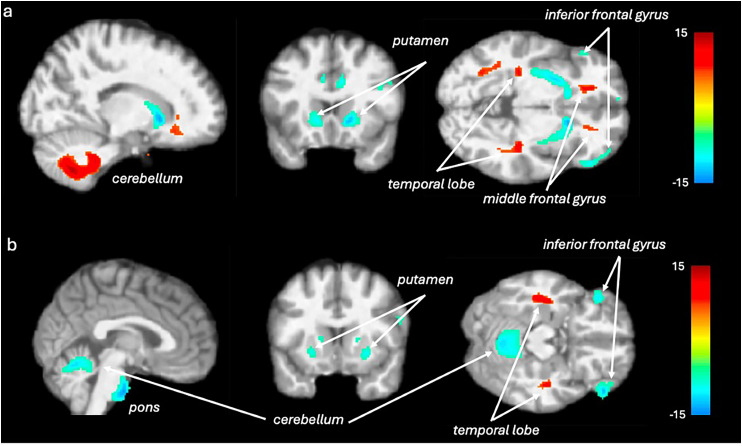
Brain regions with significant metabolic differences in MSA patients compared with healthy controls detected by SPM analysis of FDG-PET scans. a) Normalized glucose metabolism in the MSA-P patients decreased (blue) in the bilateral putamen, inferior frontal gyrus and anterior cingulate, and increased (red) in the bilateral temporal lobe, middle frontal gyrus and posterior lobe of the cerebellum compared to healthy controls (*p* < .001 uncorrected at peak-level). b) Normalized glucose metabolism in the MSA-C patients decreased (blue) in the vermis of the cerebellum, the pons, the bilateral inferior frontal gyrus, and the putamen, but increased (red) in the left and right temporal lobe compared to healthy controls (*p* < .001 uncorrected at peak-level). Only the clusters that are significant (p < .05) after FWE-correction are visualized.
